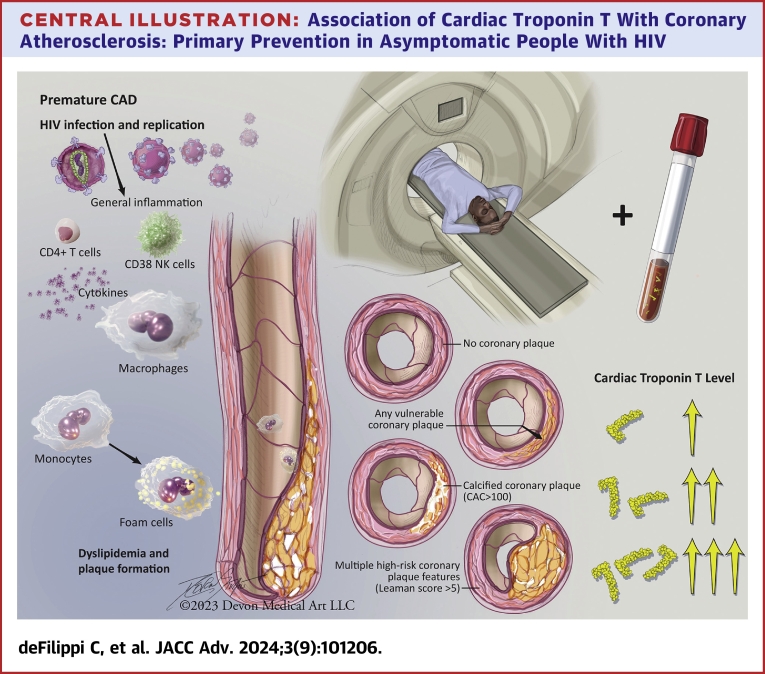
Association of Cardiac Troponin T With Coronary Atherosclerosis: Primary Prevention in Asymptomatic People With HIV People with HIV (PWH) have an increased prevalence of coronary atherosclerosis including higher risk plaque features measured by coronary CT angiography (CCTA). Levels of circulating cardiac troponin T measured with a high sensitivity assay are independently associated with the prevalence of coronary plaque, particularly with plaque features associated with higher risk for major adverse cardiac events. Abbreviation as in Figure 1.
